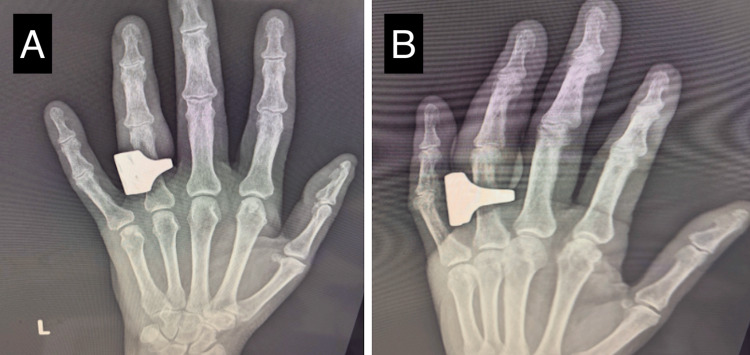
X-ray images of the patient’s right hand with the embedded ring Radiograph of the right hand depicting ring embedment, unremarkable for fractures or crepitus. A: posteroanterior; B: oblique
